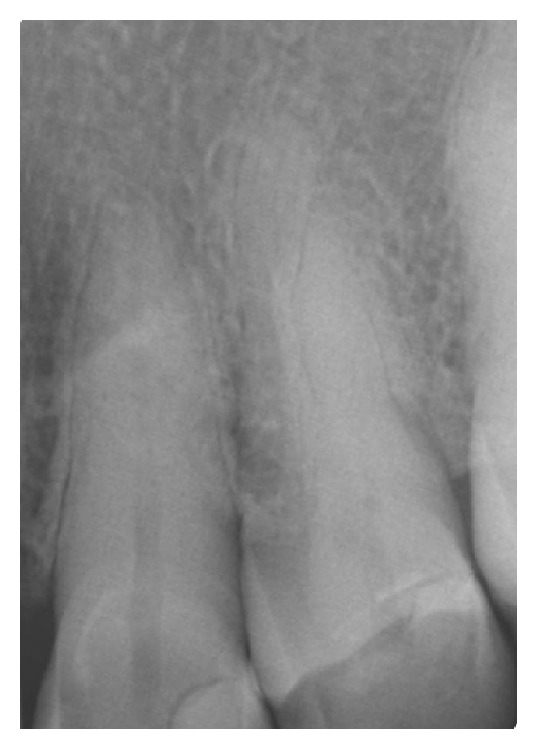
Intraoral radiograph of the PA.
